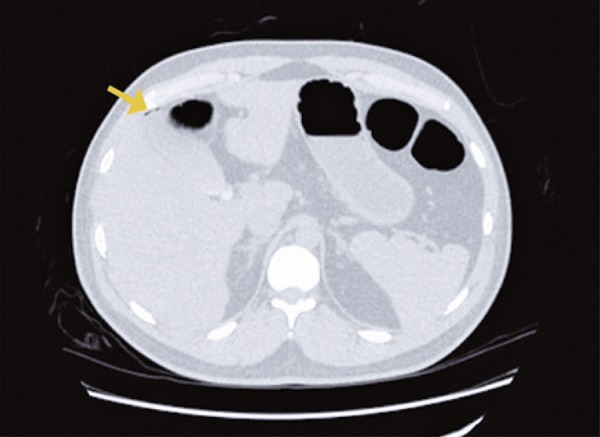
Axial computed tomography showing minimal pneumoperitoneum (arrow)
